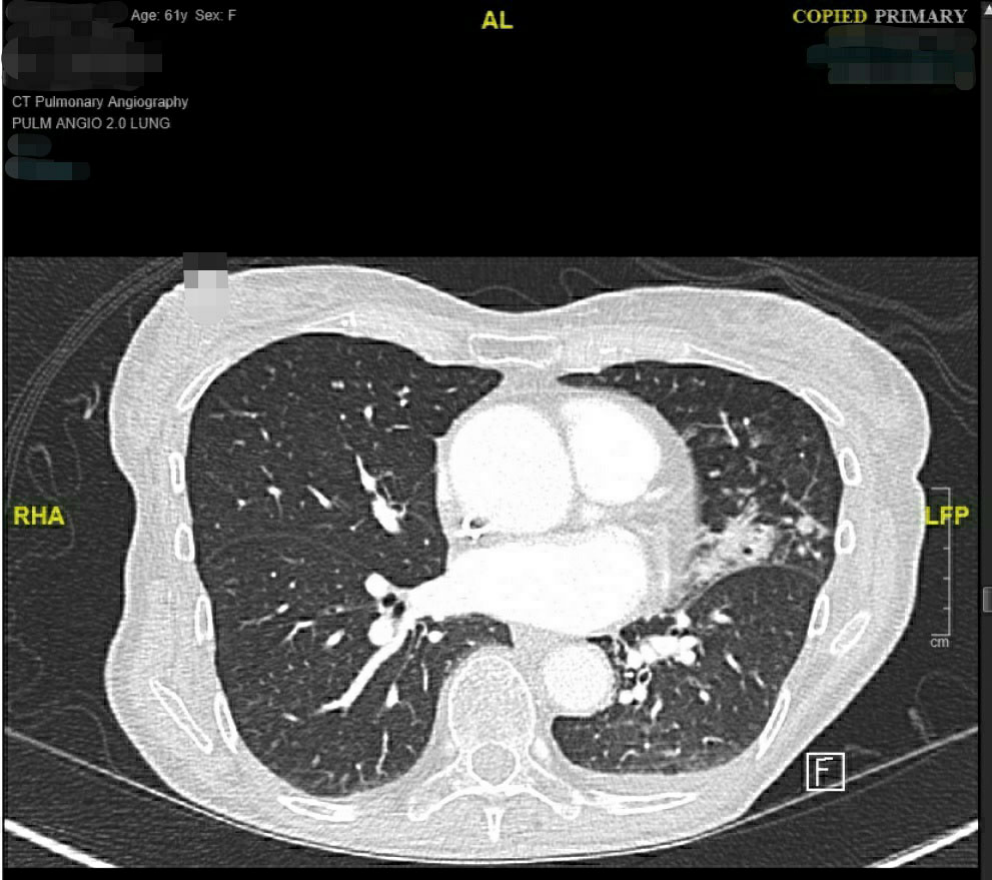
Figure 1. CT image of MAC-I infection.
